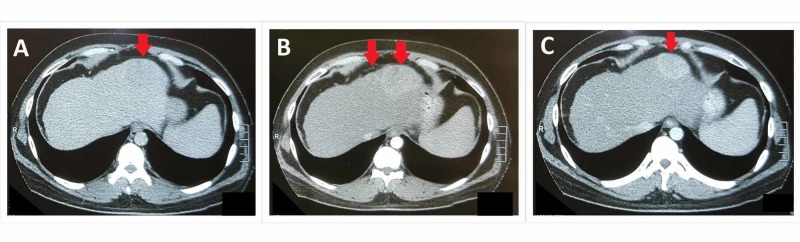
Pre-TACE Triphasic CT abdomen showing plain (A), arterial (B), and venous (C) phases of study There are two lobulated arterially enhancing (B) lesions (larger lesion measures 8.5 x 5.1 x 5.3 cm, smaller lesion measures 1.5 x 1.2 cm) in the left lobe of liver showing washout on the venous phase (C). The liver has irregular margins. CT: computed tomography; TACE: transarterial chemoembolization
